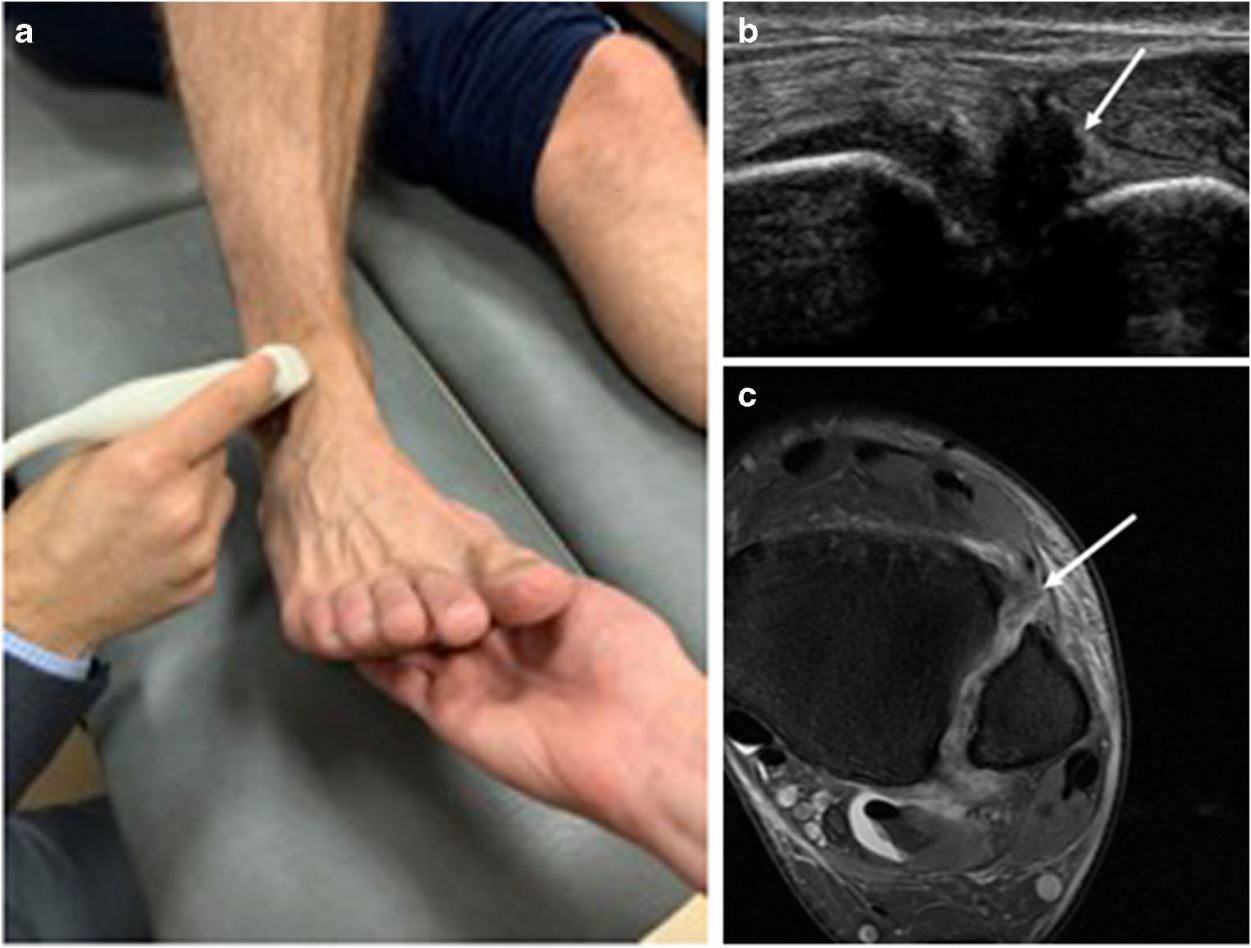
Probe position and (US/MR) imaging findings for the anterior tibiofibular ligament (AITFL). Ultrasound for the diagnosis of anterior tibiofibular ligament injury. a The ultrasound probe is placed over the AITFL in the axial plane (about 1 cm proximal to the joint line). b Ultrasound findings consistent with a complete tear of the anterior tibiofibular ligament (arrow). c Axial PD-FS image showing disruption of the anterior tibiofibular ligament, consistent with a complete tear (arrow)
